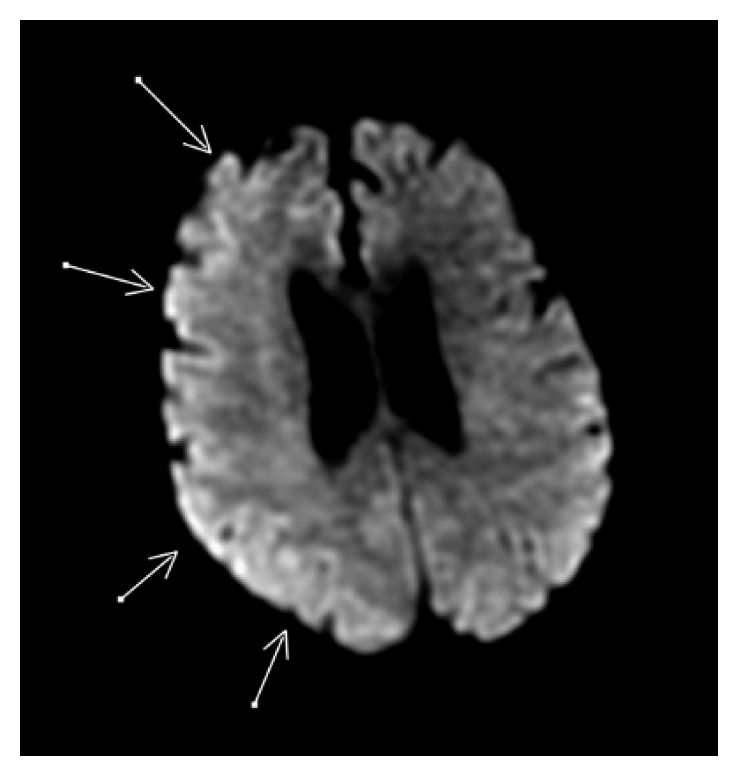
MRI with DWI showing diffuse involvement of the frontoparietal region.
